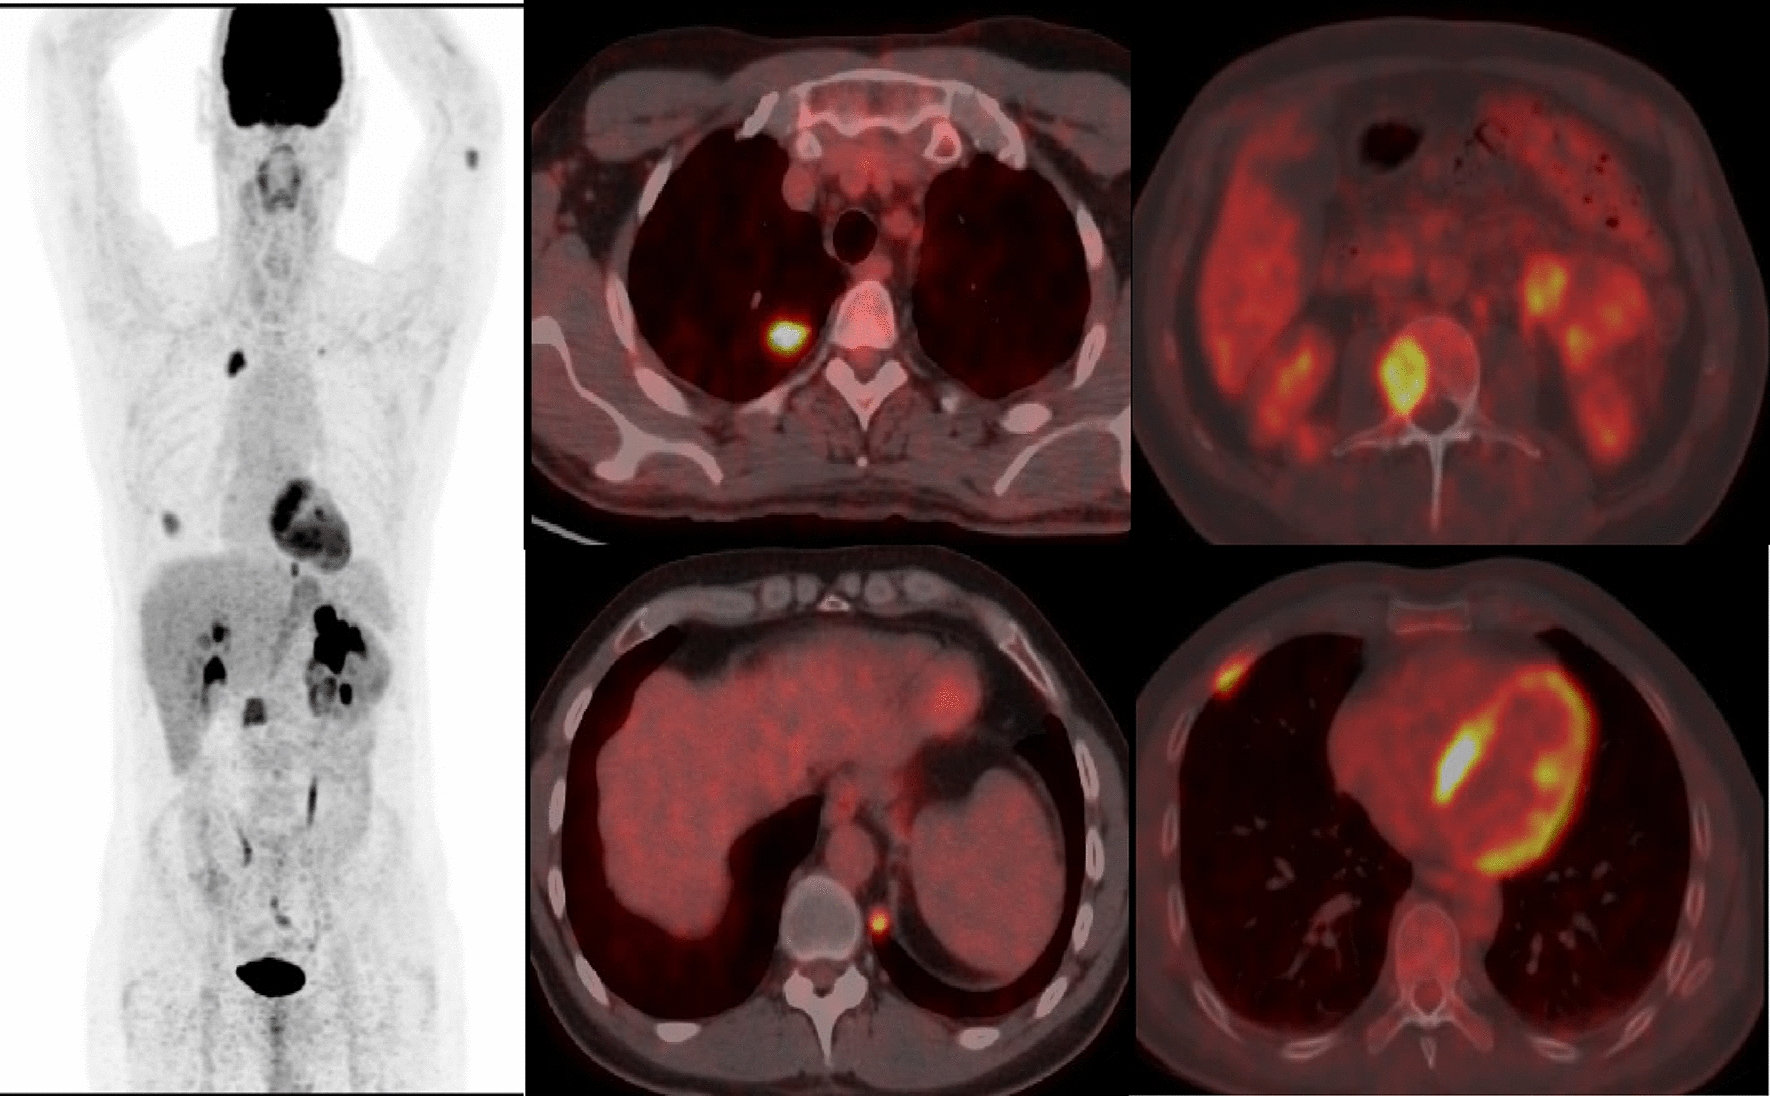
Whole-body MIP and axial fusion images from an 18F-FDG PET/CT peformed for initial staging. FDG left renal mass is seen, representing the known renal cell carcinoma. Intense uptake is seen with the previously visualized lung nodules in the right upper and left lower lobes, as well as additional FDG avid lytic osseous lesions in the right anterior 5th rib and the L3 vertebral body, consistent with distant metastases
